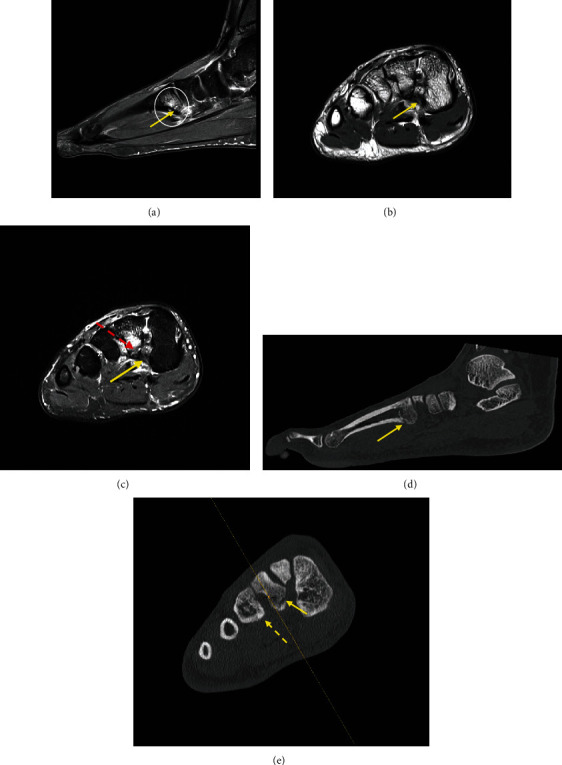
(a) Sagittal proton-density-weighted, fat-suppressed sequence (PDFS) at the level of the second metatarsal. There is localized marrow oedema signal centered at the base of the second metatarsal plantar margin (circled), with adjacent soft tissue oedema. The inferior cortex is thickened, with presence of intracortical high signal consistent with osteitis (arrow). Periosteal oedema present. The intracortical signal traversing the base of the second metatarsal is suspicious for an undisplaced fracture. Axial sequences at the level of the second metatarsal base in proton density (PD) (b) and PDFS (c) show thickening of the plantar Lisfranc ligament (arrow) which returns intermediate signal but is otherwise intact, consistent with grade II sprain or partial tear. Undisplaced fracture at the metatarsal base as shown (dashed arrow). Sagittal (d) and axial (e) CT at the similar level demonstrates the avulsion fracture at the base of the second metatarsal at the site of the plantar Lisfranc ligament insertion (arrow). There is also cortical thickening of the border of the third metatarsal base at the site of plantar Lisfranc attachment (dashed arrow).
